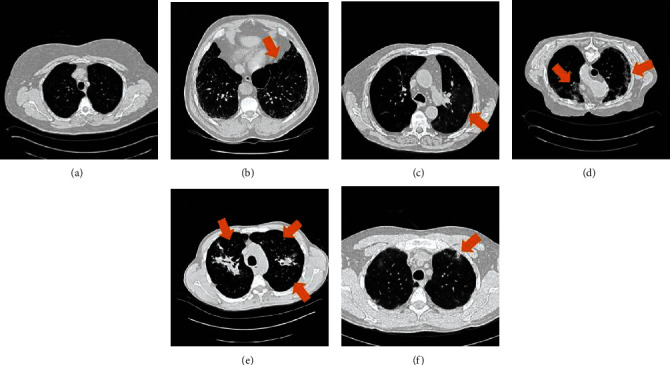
Few samples of CT image slices in the ILD dataset for six lung tissue forms [46].
